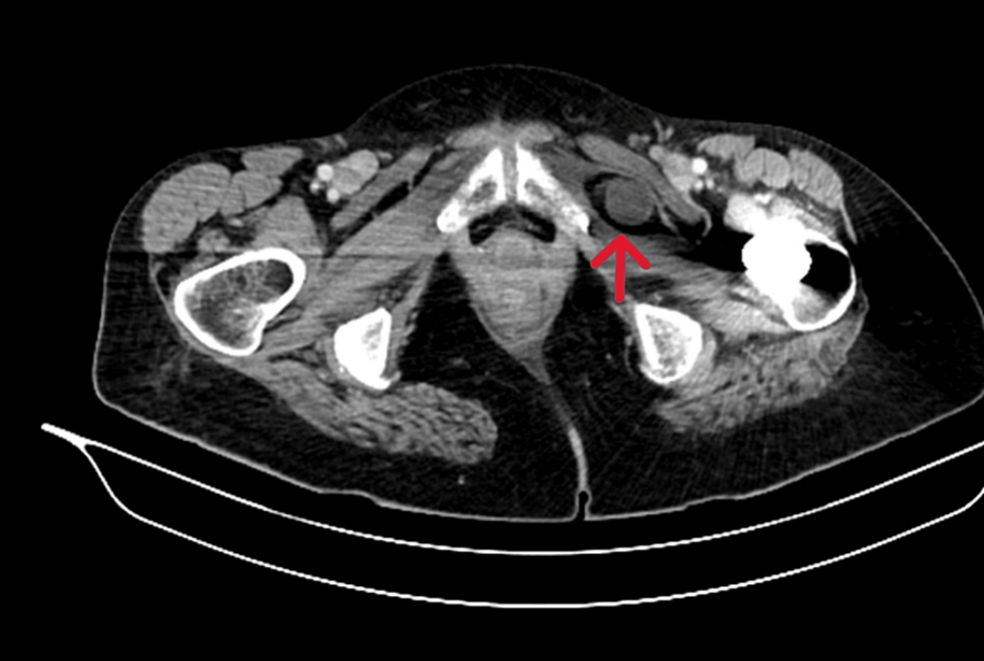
Axial CT scan of the abdomen showing an obturator hernia on the left side (marked by arrow).
